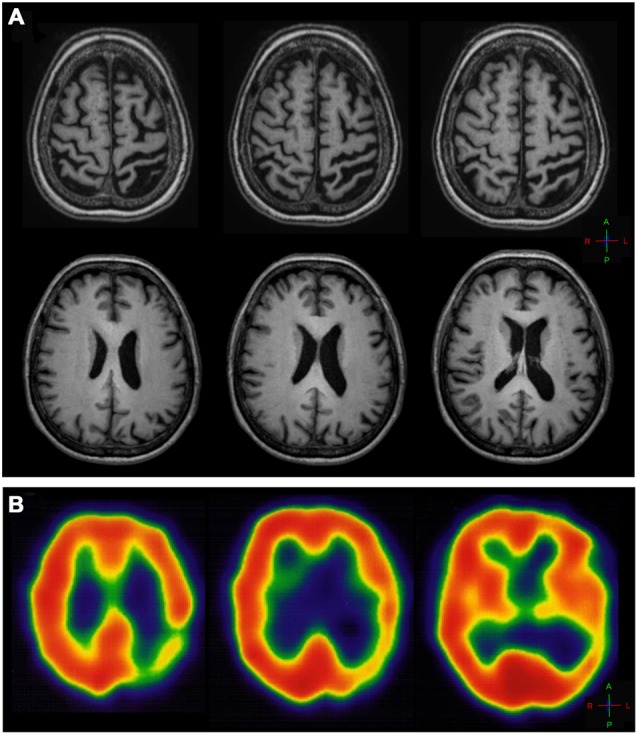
(A) T1-weighted axial MR image of the brain showing diffuse cortical atrophy and ventriculomegaly in the left hemisphere, marked in the left central sulcus and precentral gyrus and more prominent on the left post-central gyrus and parietal-posterior area. (B) SPECT axial slices of thechnetium-99m-HMPO brain perfusion scan showing deficient cerebral perfusion in the left posterior parietal-occipital cortex.
